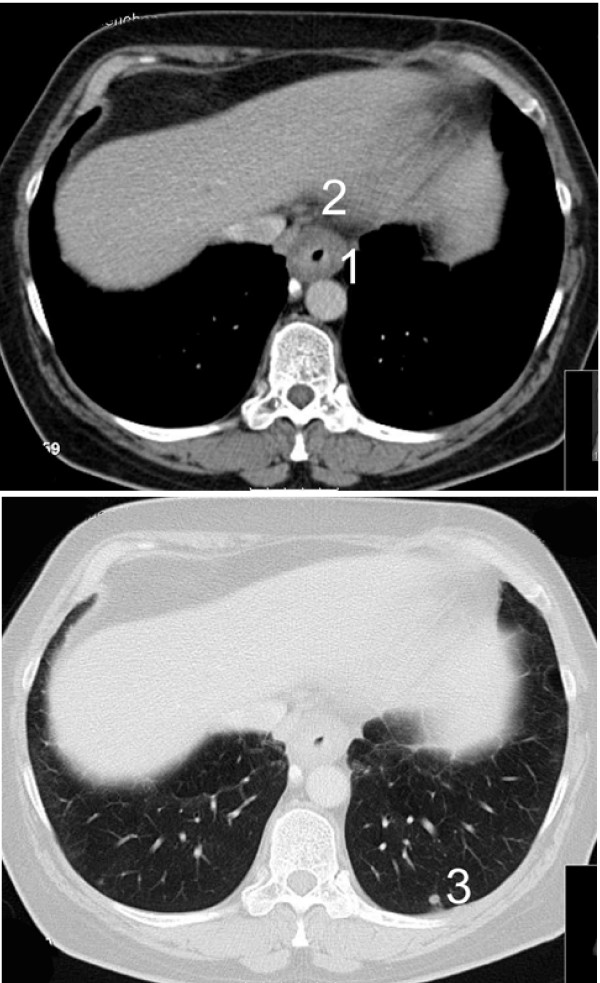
CT scan showing a circular oesophageal cancer (1.) with paraoesophageal lymph node involvement (2.) and small sarcoid lesions of the lung (3.).
